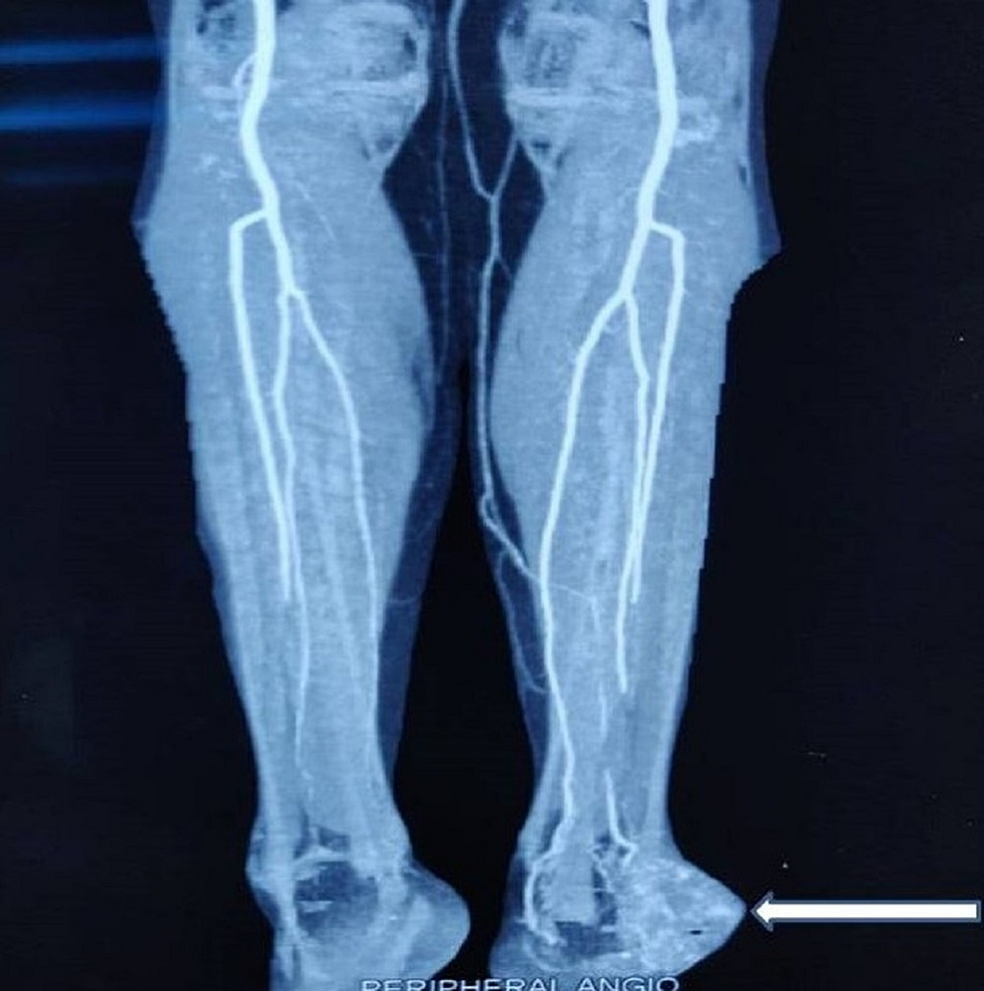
CT angiogram showing the calcaneal branches of peroneal artery feeding the tumor CT: Computed Tomography
